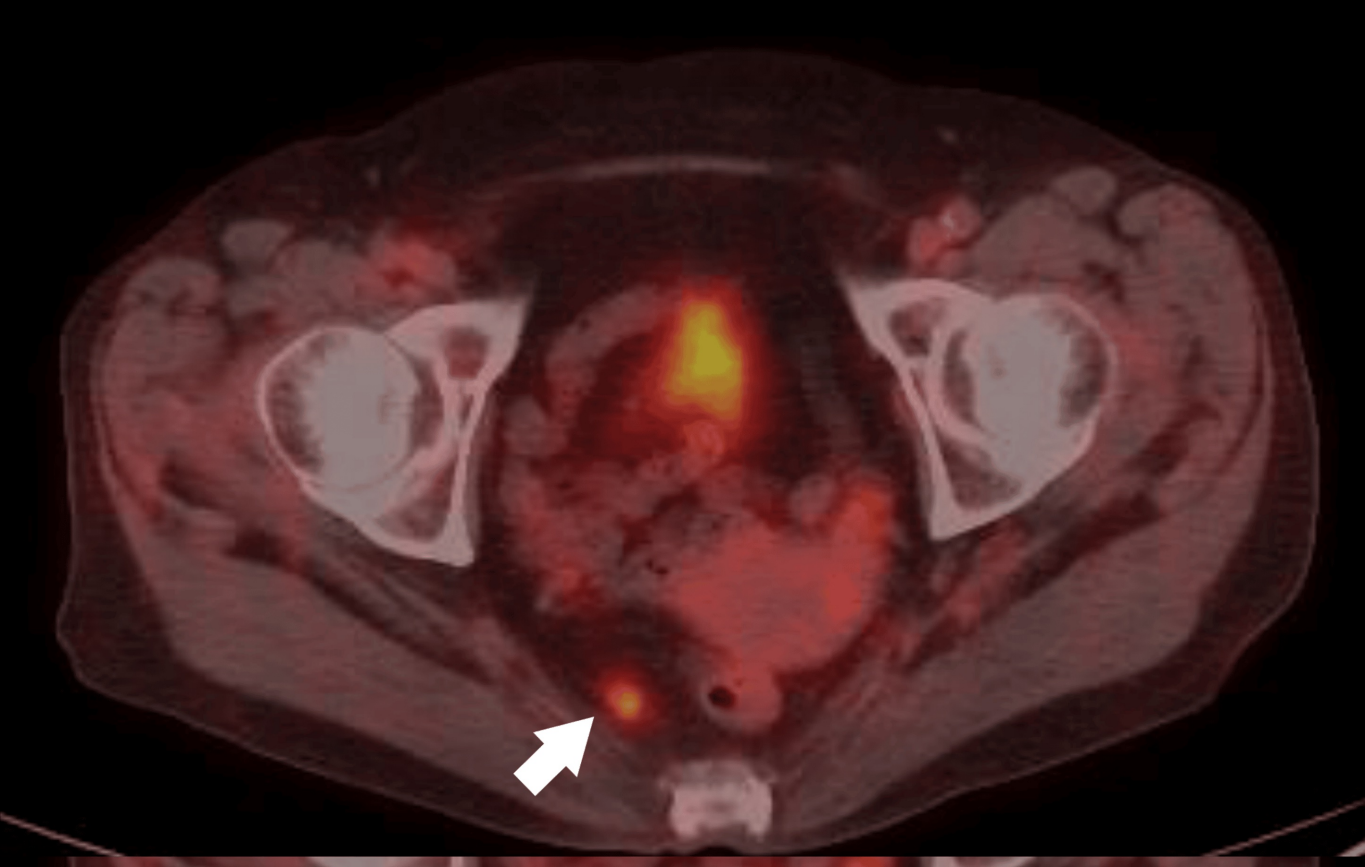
Fluorodeoxyglucose (FDG)-PET/CT image showing uptake in a perirectal lymph node (SUVmax: 3.67, arrow).
